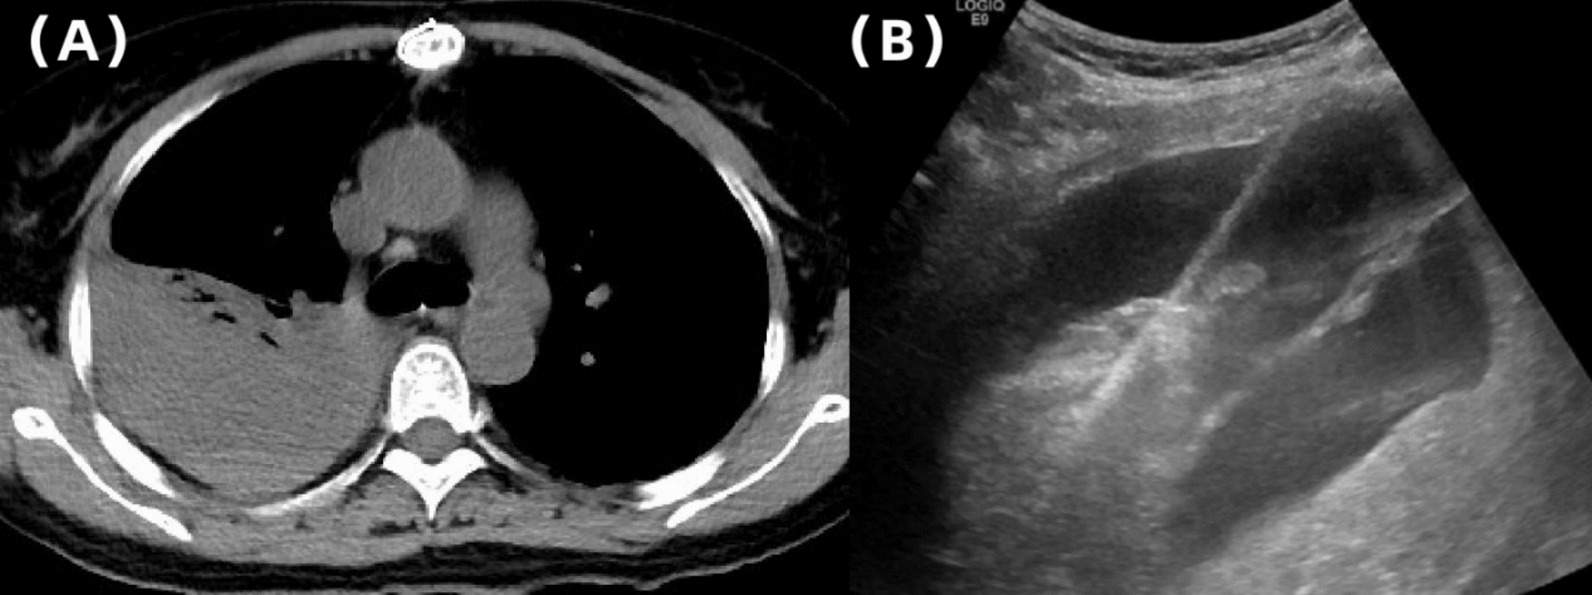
A Reexamination of chest computed tomography following transarterial embolization revealed a massive hemothorax on the right side. B A thoracic drain was inserted under ultrasound guidance
